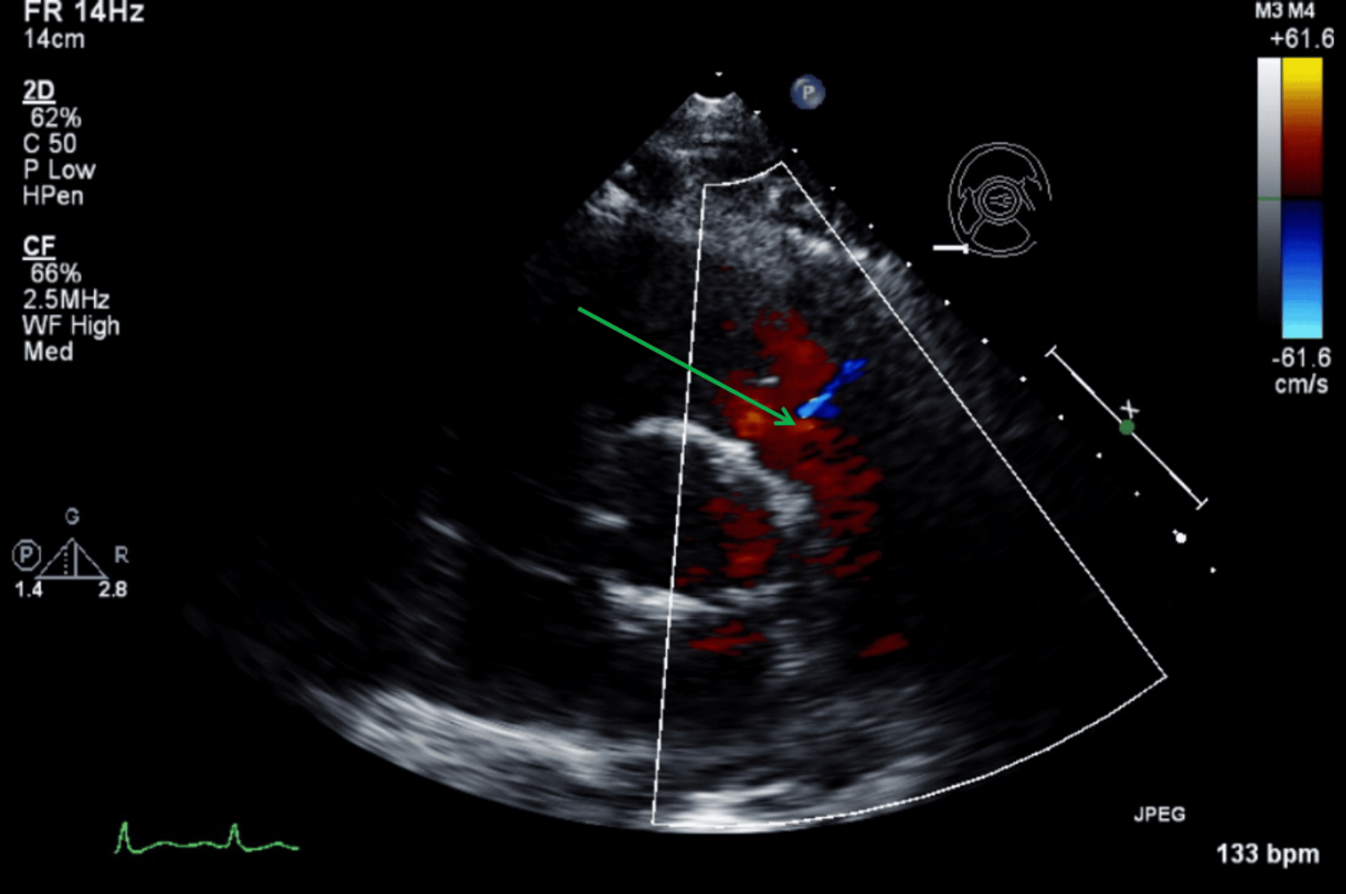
TTE parasternal short-axis view with color Doppler during diastole. The green arrow shows findings of mild pulmonic valve regurgitation with color Doppler. TTE: transthoracic echocardiography
